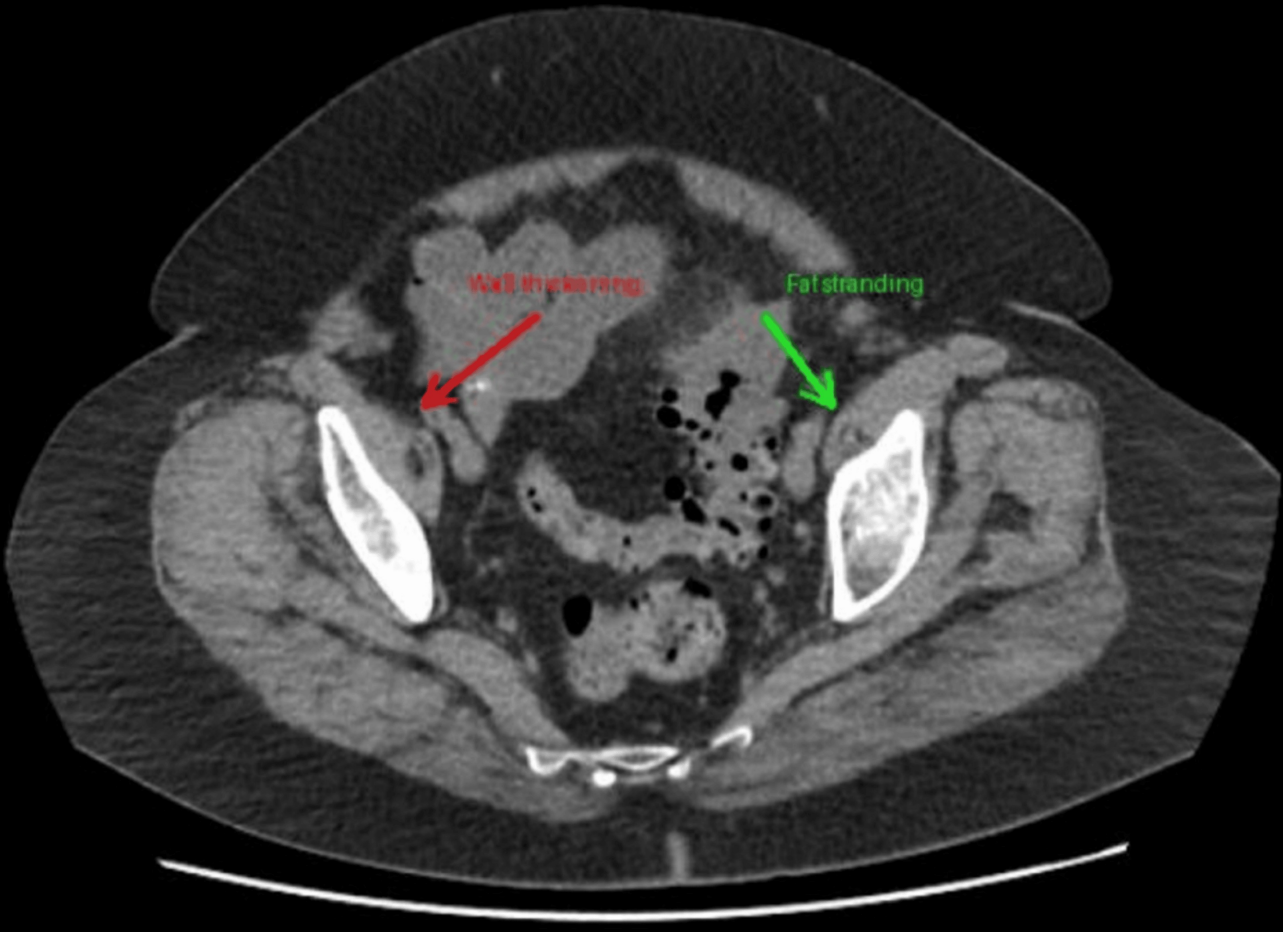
CT scan showing acute diverticulitis with characteristic bowel wall thickening and pericolic fat stranding. An axial CT scan showing acute diverticulitis with characteristic bowel wall thickening (red arrow) and pericolic fat stranding (green arrow). Original image from an anonymized patient in this study cohort. Image Credits: Maidstone and Tunbridge Wells NHS Trust, used with permission (relevant findings discussed in [4,10,11]).
